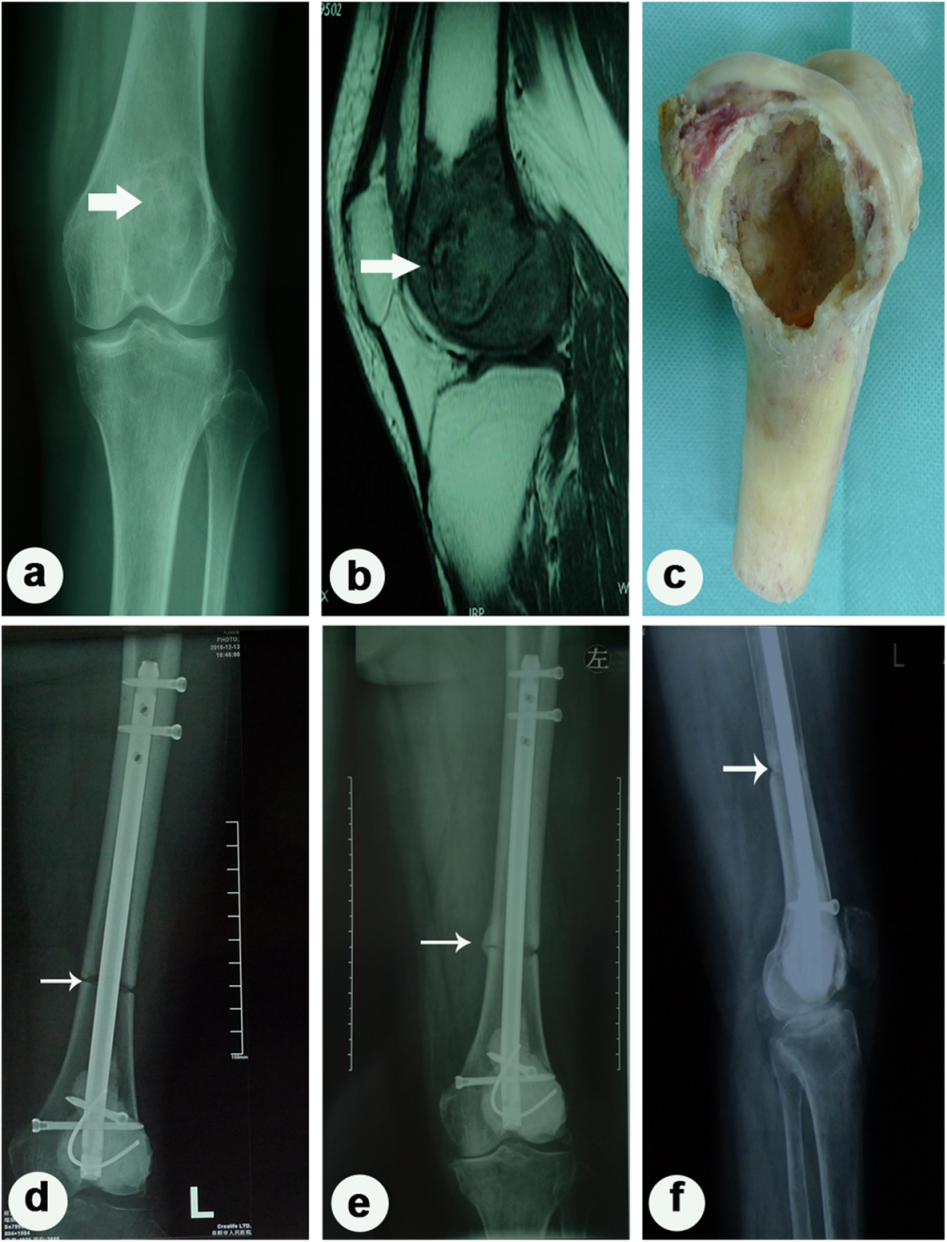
Case presentation I. Case 43: A 19-year-old man was diagnosed as having osteosarcoma in the distal of his left femur and was treated with wide resection and inactivated autograft using alcohol. a Plain radiography before surgery. b MRI before surgery. c Inactivated autograft by alcohol in surgery. d Three months after surgery. e One year after surgery. f Two years after surgery
